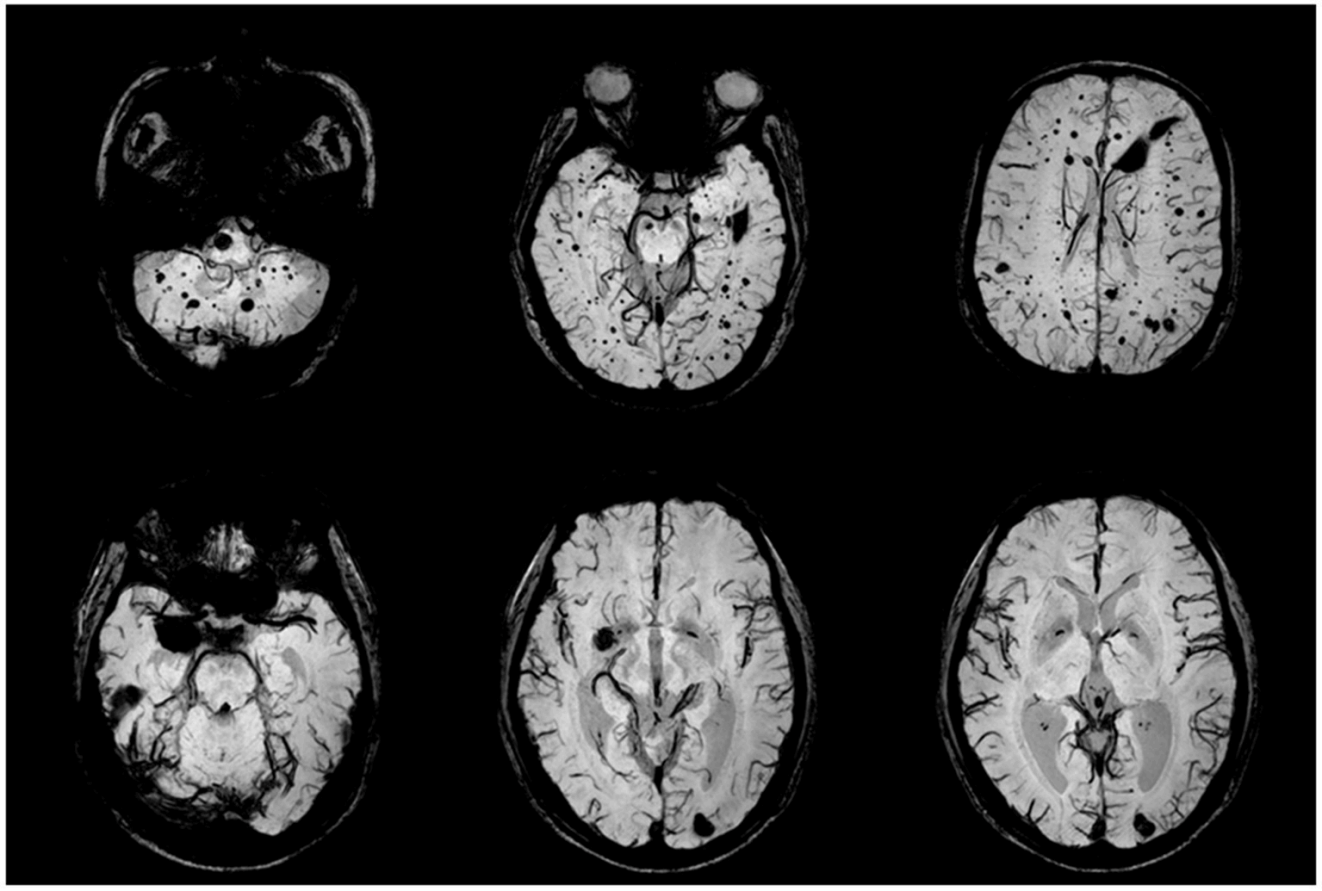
CCM lesions in Family One detected by SWI-MRI. The proband's SWIs (II:1) revealed dot and patchy low intensity lesions on temporal lobe, cerebellum and thalamus (upper row). SWI (II:2) showed 2 popcorn-like lesions in left occipital region and right temporal pole, which were very dark, suggesting chronic bleeding.
